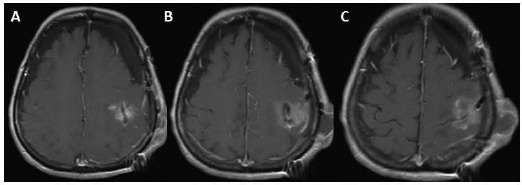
A, B, C (Table 1: Case 2, Cavernoma): Gadolinium-enhanced T1 weighted axial magnetic resonance image scans taken at the postoperative period showing no residual lesion, but there is some postoperative hyperintense changing due to gliosis at the site of the operation. Also, it is noteworthy that we excised the trichilemmal cyst, so it is not seen on the scans*.
